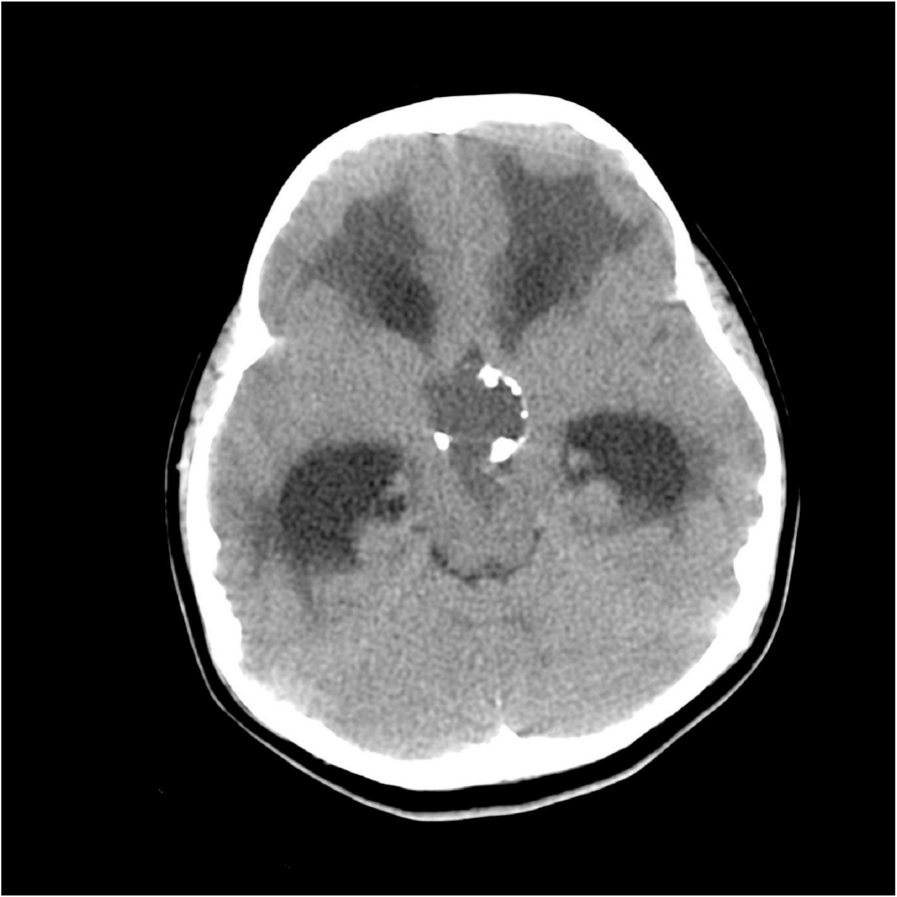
Preoperative CT of the head with contrast (16 June 2016). The tumor, 25x40x31 mm (I-I, a-p, c-c) in size, compresses onto the 3rd ventricle and interventricular foramina. Ventricular system of the brain distended (Evans ratio 44%), symmetrical, with the signs of a transependymal oedema
